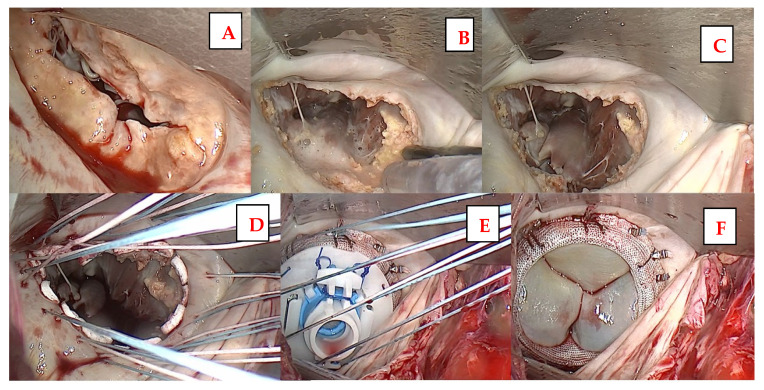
Microinvasive fully endoscopic implantation of the MITRIS RESILIA mitral valve prosthesis. (A): Native mitral valve with a combined pathology (regurgitation and stenosis), (B): ultrasonic decalcification of the mitral annulus after excision of the native leaflets, (C): result after decalcification, (D): insertion of the valve sutures, (E): implantation of the MITRIS RESILIA mitral valve, (F): final result of the implanted MITRIS RESILIA mitral valve.
